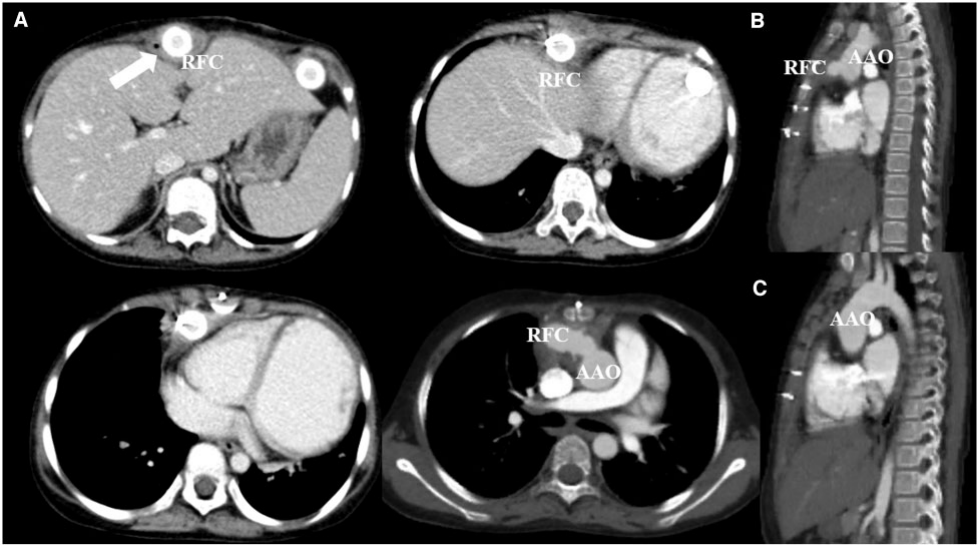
Contrast-enhanced computed tomography performed on Day 729. (*A*) Axial view showing fluid retention around the cannulation site (white arrow), which is suggestive of infection. No signs of pseudoaneurysm are observed at this time. (*B* and *C*) The sagittal view is shown. The ascending aorta is intact.
